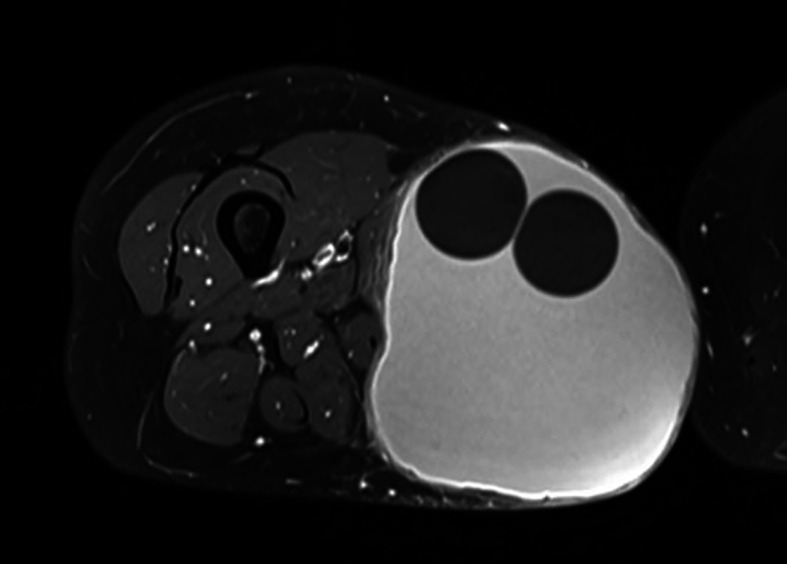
MRI appearance of the cyst showing daughter cysts in its center and a subtle peripheral enhancement after gadolinium injection.
